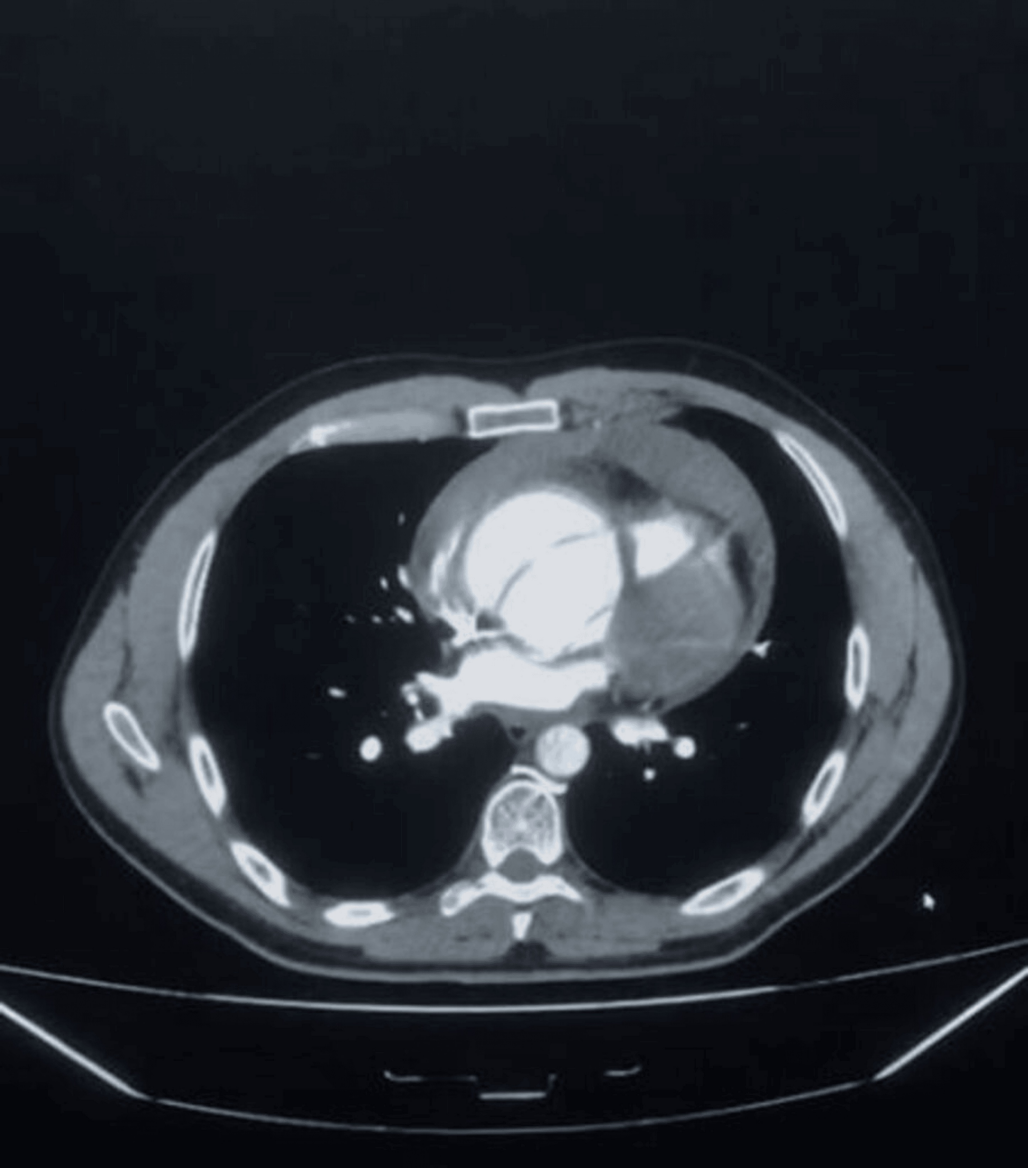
CT with contrast showing aortic aneurysm
